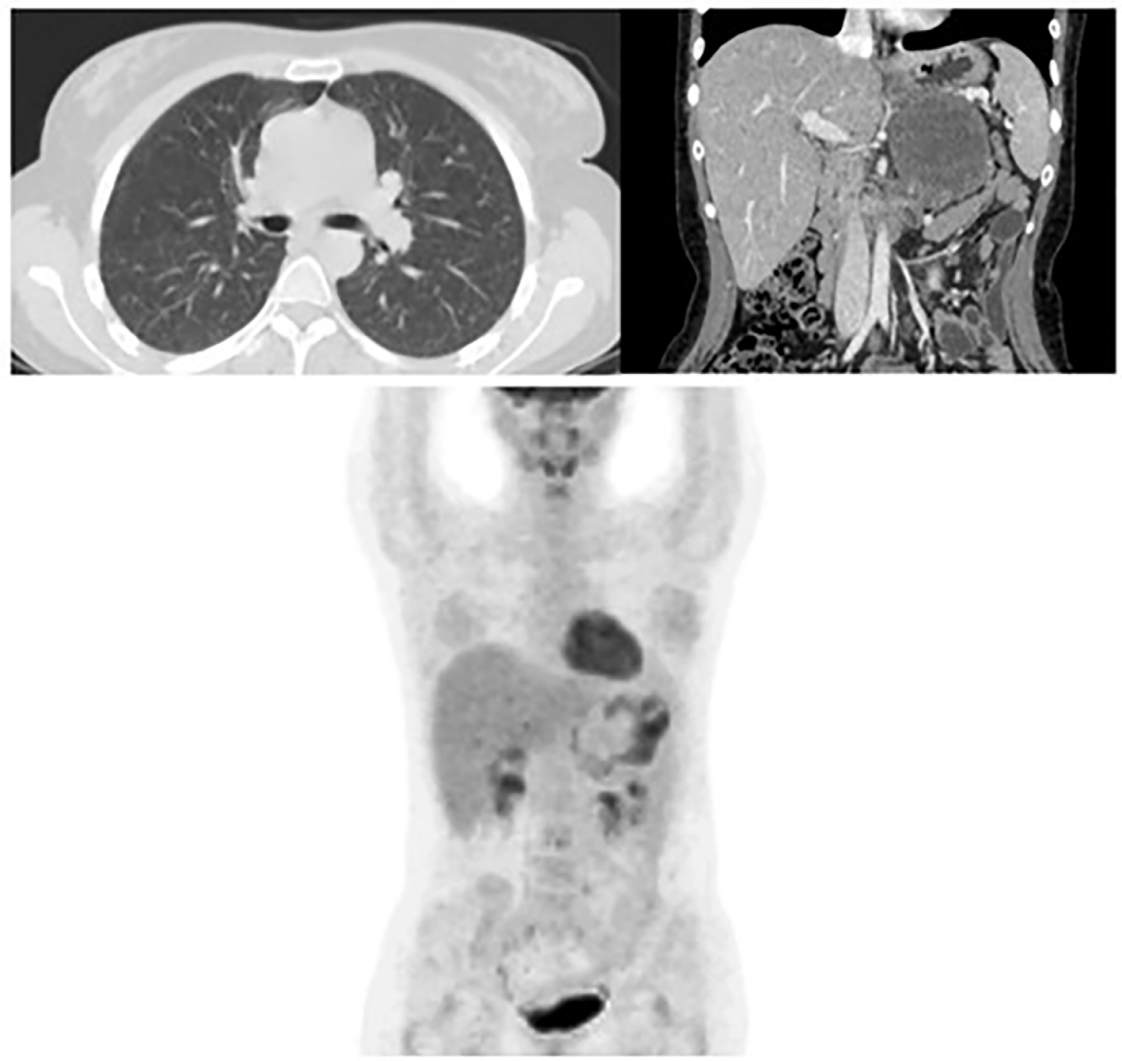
Complete response of the bilateral lung metastases and reduction of the primary adrenal lesion at the CT Scan after 8 cycles of temozolomide. FDG PET confirming the complete response of lung lesions and a small peripheral area of FDG uptake at the residual adrenal mass. (September 2020).
